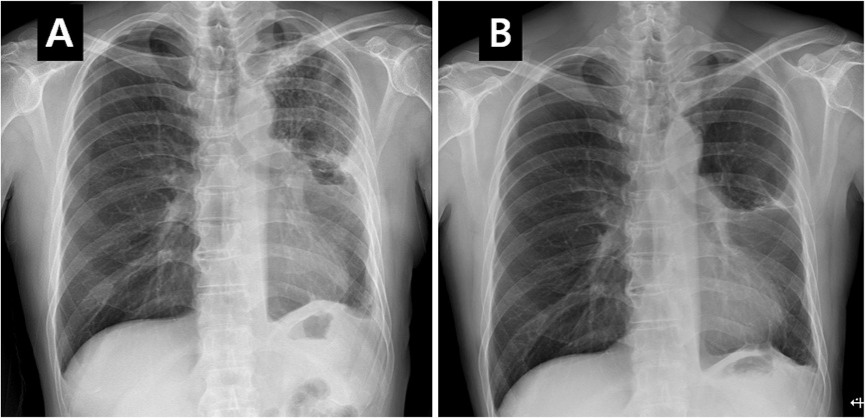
X-ray findings of resolving the acute pneumonitis. A follow-up chest X-ray showed a markedly decreased pulmonary infiltrations so as the remaining chest tube was removed (A). The patient was discharged twenty-six days after the segmentectomy and followed- up for four months without any respiratory symptoms (B).
